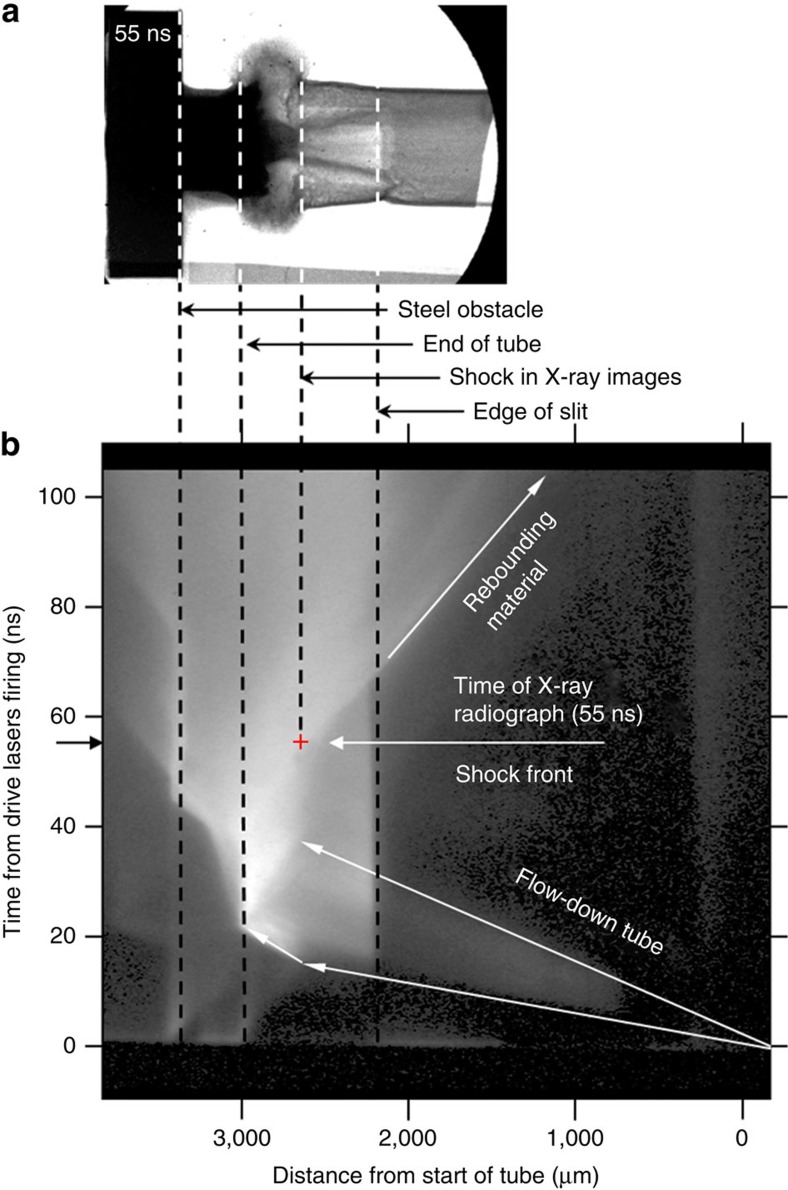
Streaked optical image of the flow and shock evolution. (a) An X-ray image taken from Fig. 2 aligned with the streaked optical image in (b). Optical diagnostics were fielded in the experiment to record the self-emission from the hot plasma along the entire length of the tube. An imaging system directed the light emitted by the interacting plasma to the streak camera. The streak camera had a spatial field of view of ∼4 mm and thus the entire tube and obstacle were visible, except the region shielded by the copper cone. An imaging line aligned with the central axis of the tube (along the slit cut-out in the tube), in the horizontal plane, was then streaked in time over ∼60, 100 or 250 ns as required. This provided a temporal dependence of flow velocities in plasma. Time runs from bottom to top, distance right to left. The time evolution of the plasma can clearly be seen inside the tube. The dotted lines and overlaid radiograph show positions of parts of the target on the streaked image. There is good agreement in the position of features between the X-ray (a) and optical (b) diagnostics.
